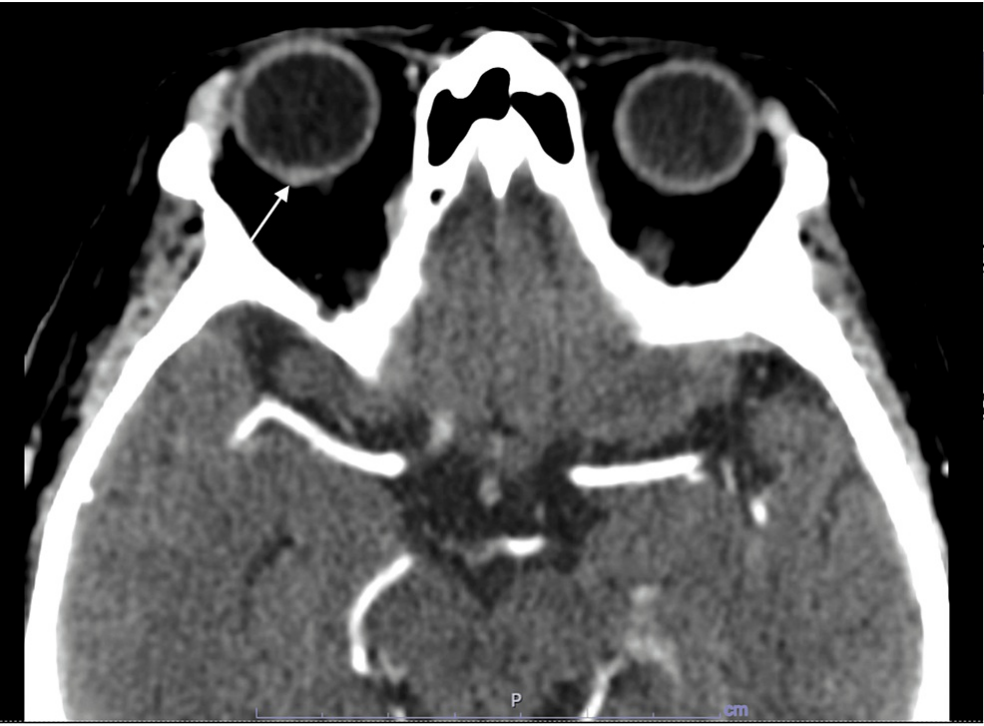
Orbit CT scan revealed a notable thickening of the choroid laterally to the optic nerve insertion. The use of contrast enhancement enhances the visibility of this distinct area of interest.
